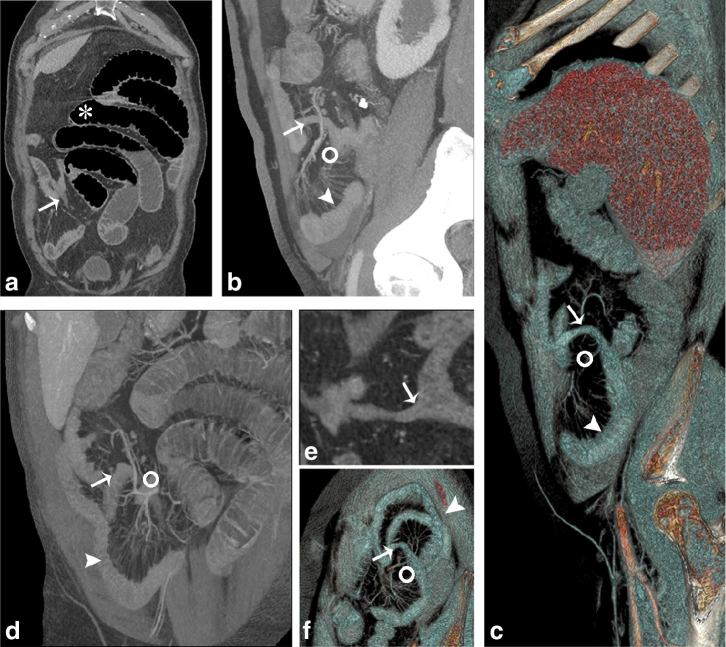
(a) Coronal reconstruction; (b) sagittal MIP reconstruction; (c) volume rendering parasagittal view; (d) oblique MIP reconstruction; (e) curved reconstruction; (f) volume rendering paracoronal view. Coronal reconstruction (a) of contrast-enhanced CT scan shows diffuse small intestine dilatation (asterisk) with multiple air–fluid levels. The transition point between the distended and the collapsed bowel loops is located at the level of a fibrous connection between the MD (arrow) and the anterior abdominal wall. A cluster of collapsed bowel loops (arrowheads; b, c, d and f) herniated throughout this orifice (circles in b, c, d and f) and configuring an internal hernia is also clearly visible. Figure (e) represents a curved reconstruction that shows the origin the MD from the ileal antimesenteric border (arrow). MD, Meckel’s diverticulum; MIP, maximum intensity projection.
